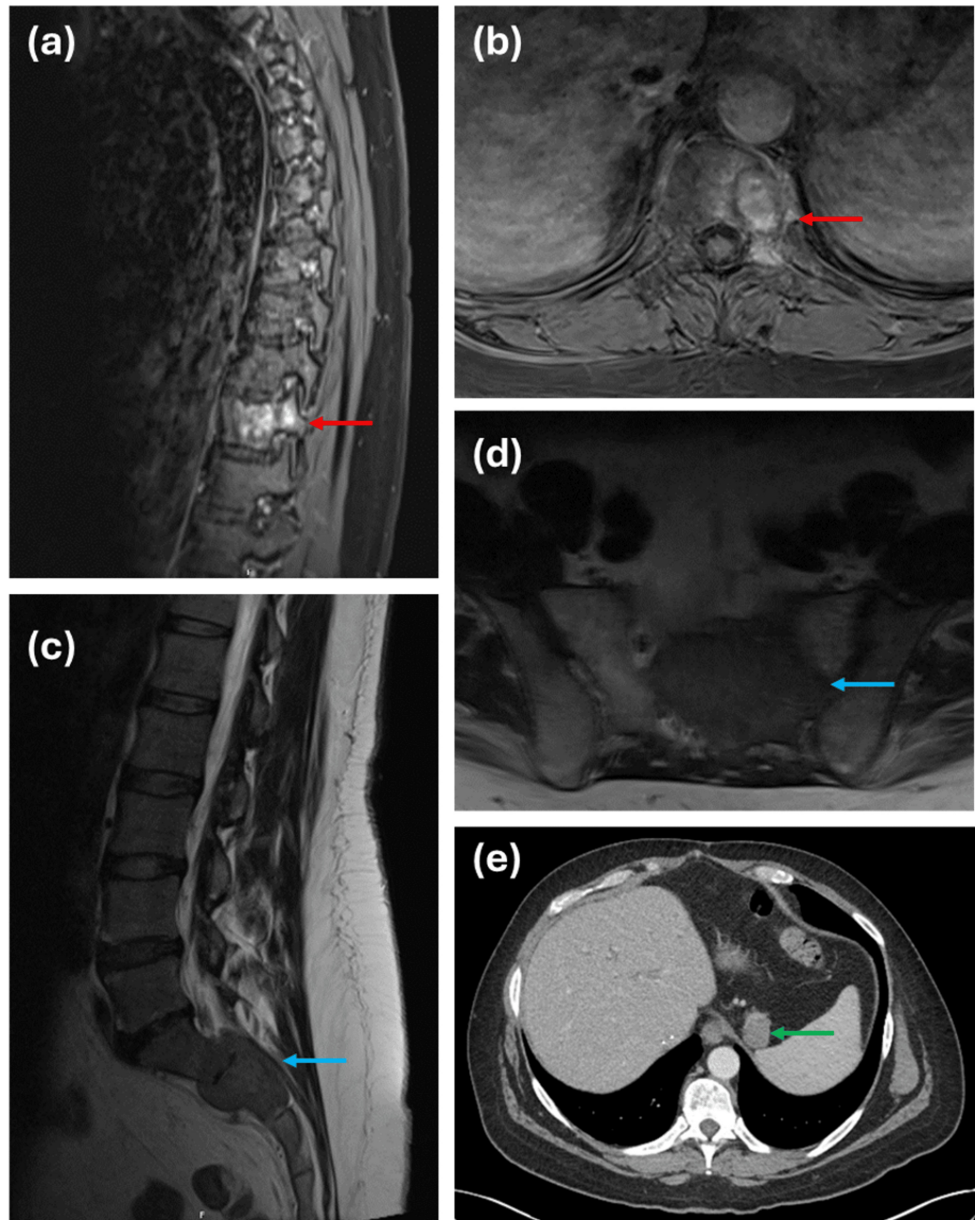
The identified lesions for (a-b) T11 and (c-d) S2, as shown on MRI, and (e) gastrosplenic lymph node, as shown on CT.
